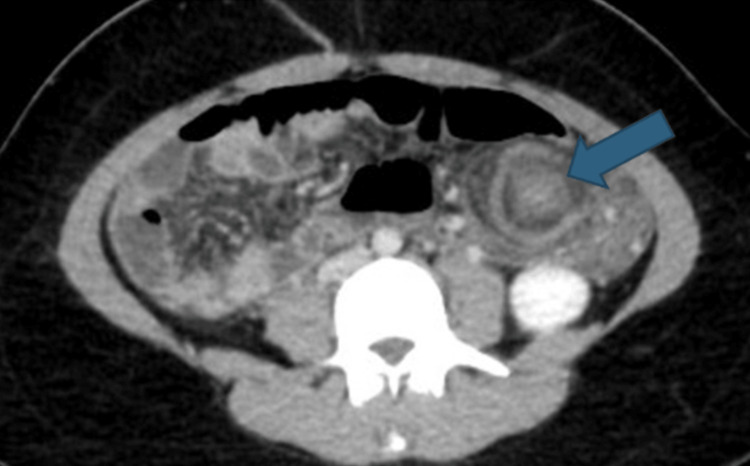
The axial plane of contrast-enhanced computed tomography images showing an enlarged spleen with a low position Blue arrow reveals a twisting of the splenic vessels “whirlpool sign”
